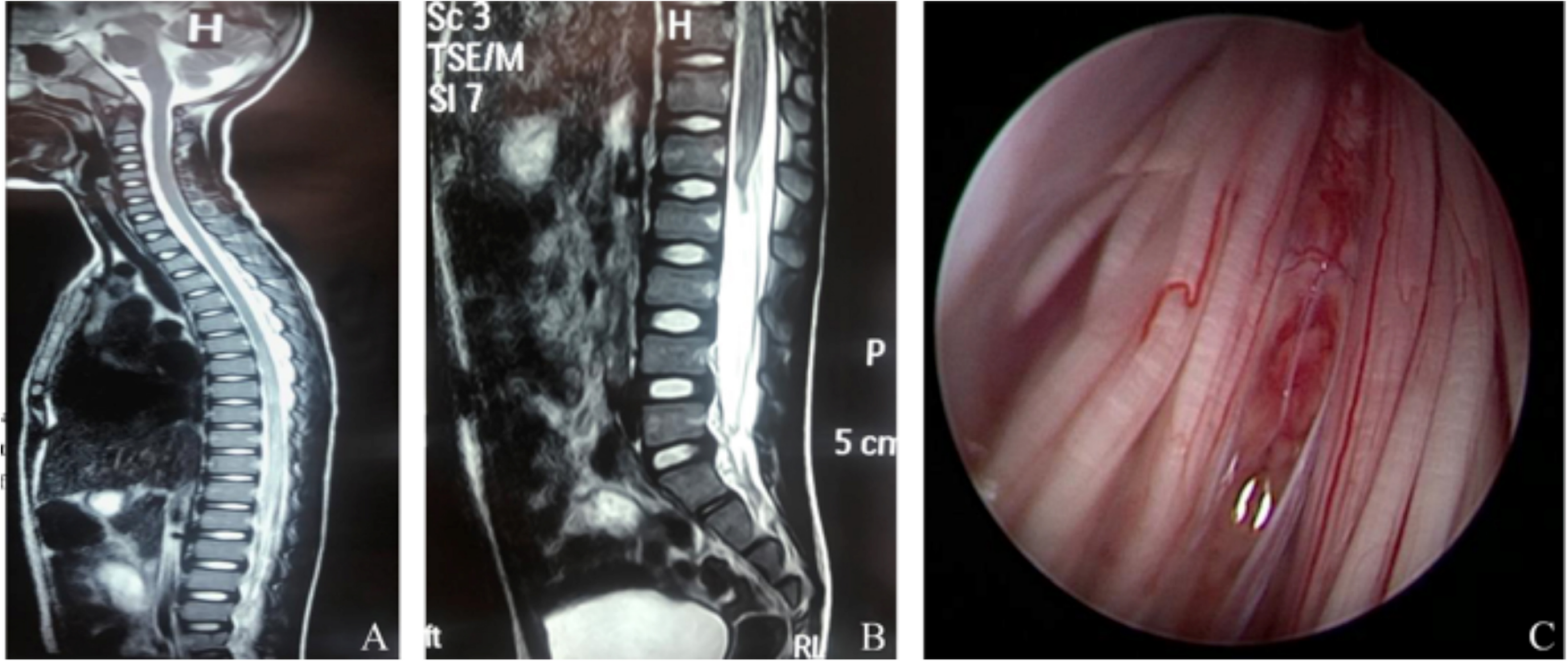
a The sagittal MRI images showed longitudinally extended diffused intramedullary patchy T2 hyperintensity at the T12-L1 level. b The conus was located at L1 level with mild swelling. c Contusion in the conus, terminal filum fibrolipoma and TFT were found in the operation
